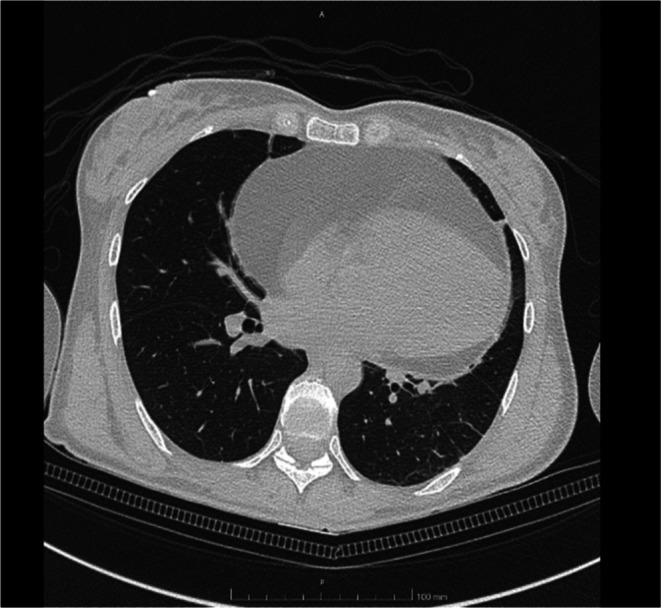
Chest‐CT—This transversa chest CT image shows a large mass (maximum diameters on the axial plane 17 × 11 cm) encasing the heart with mature fat aspect (low density–HU–of the tissue surrounding the heart).
